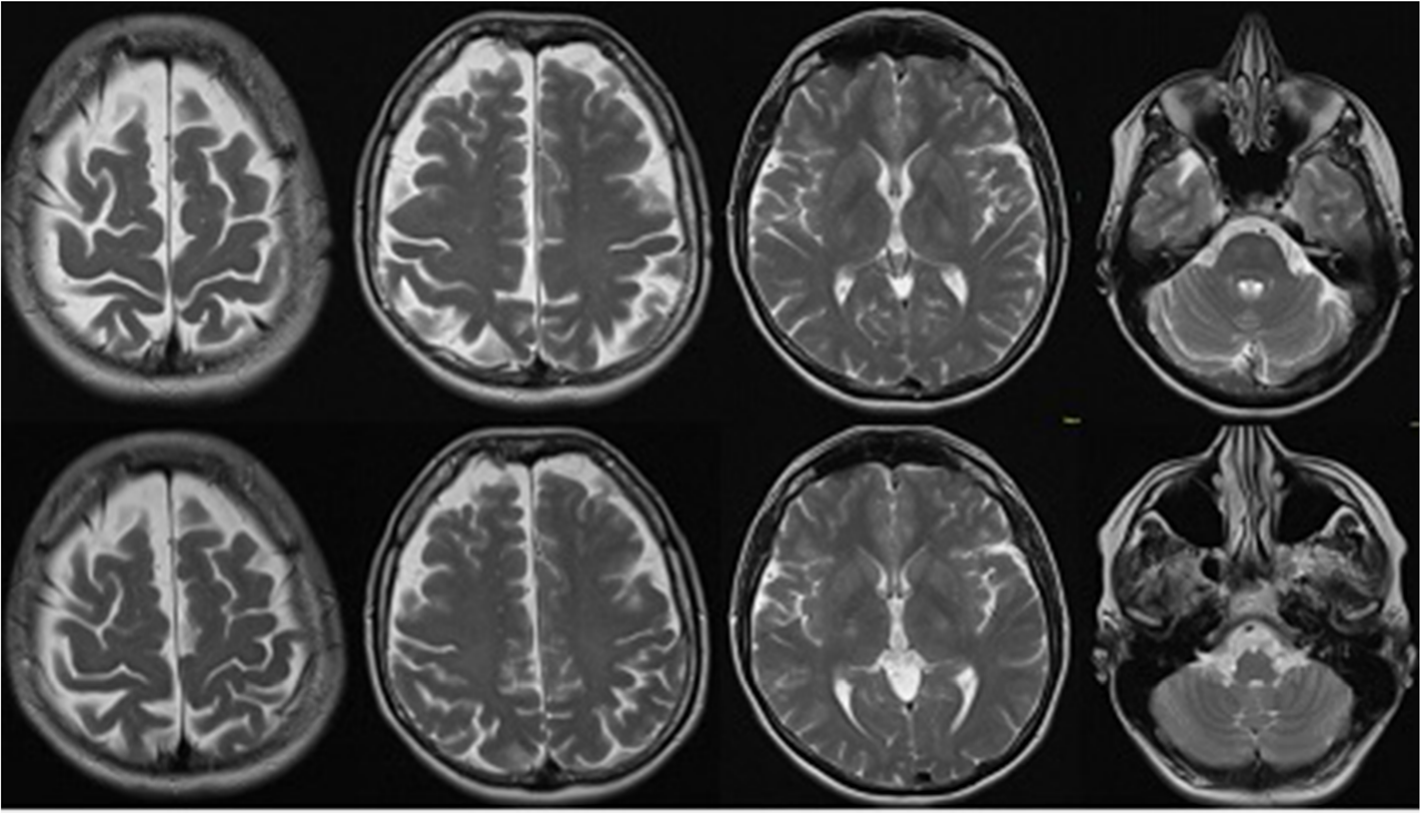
Magnetic resonance imaging (MRI; T2 weighted axial scans) before steroid treatment depicted unspecific supratentorial white matter lesions and frontal accentuated brain atrophy (upper row). Follow-up MRI 7 months after the start of therapy showed no changes (bottom row)
